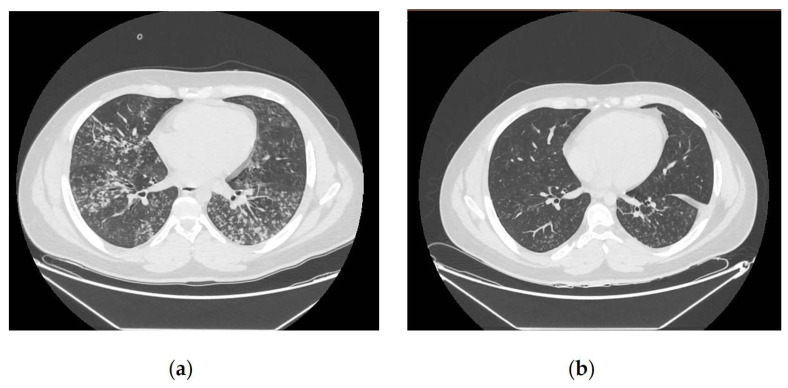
Chest CT findings at admission and follow-up: (a) axial CT images obtained on admission show diffuse centrilobular micronodules involving the middle lobe, lingula, and basal segment of the left lower lobe, consistent with atypical pneumonia; (b) follow-up CT performed on day 13 demonstrates marked reduction in micronodular opacities and partial re aeration of previously affected regions.
